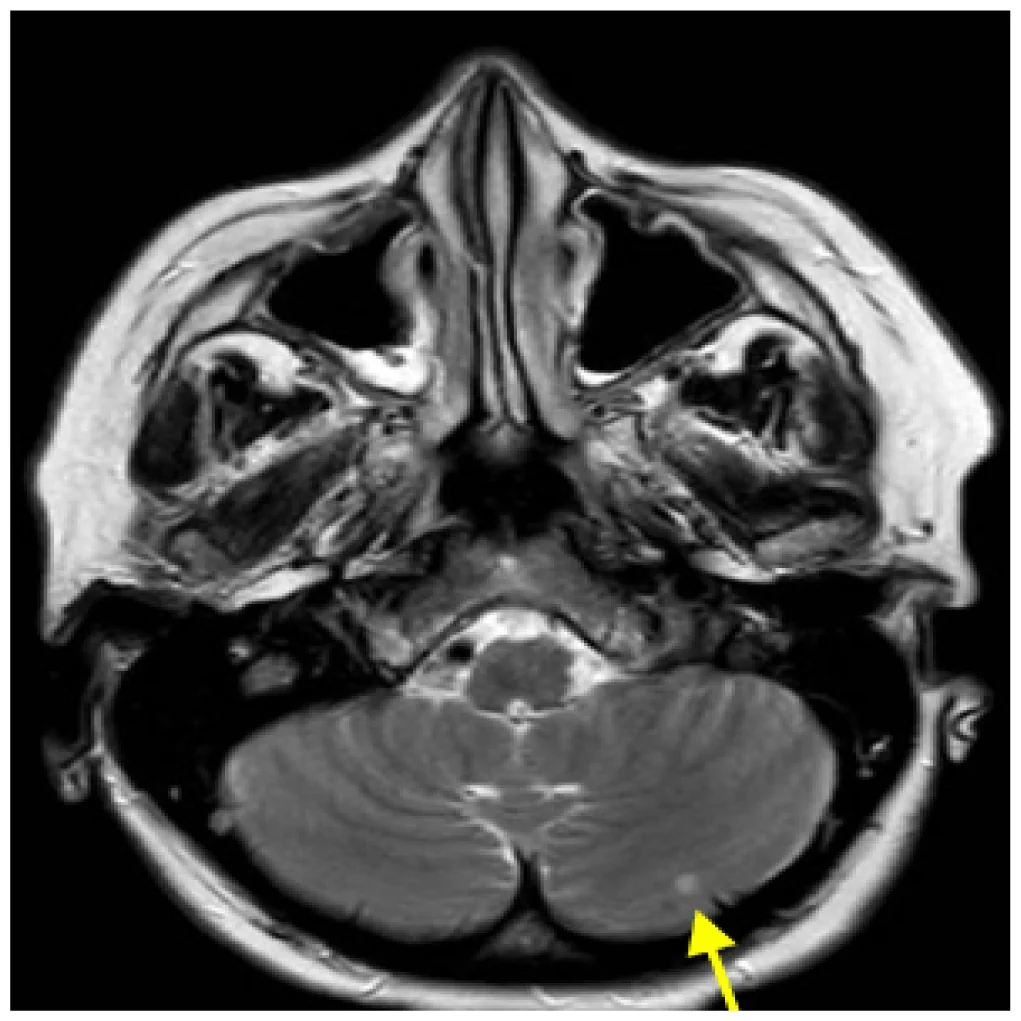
Impression: No foci of restricted diffusion to suggest acute or recent infarction. No abnormal foci of susceptibility artifact or other signal to suggest acute intracranial hemorrhage or abnormal hemosiderin/mineral deposition.	Impression: Two tiny sites of signal abnormality within cerebellum (one in each hemisphere as above), likely tiny acute or subacute lacunar infarctions and new from the recent prior.	Impression: A small focus of restricted diffusion and T2/FLAIR hyperintense signal has developed within the left cerebellum compatible with acute ischemia.
